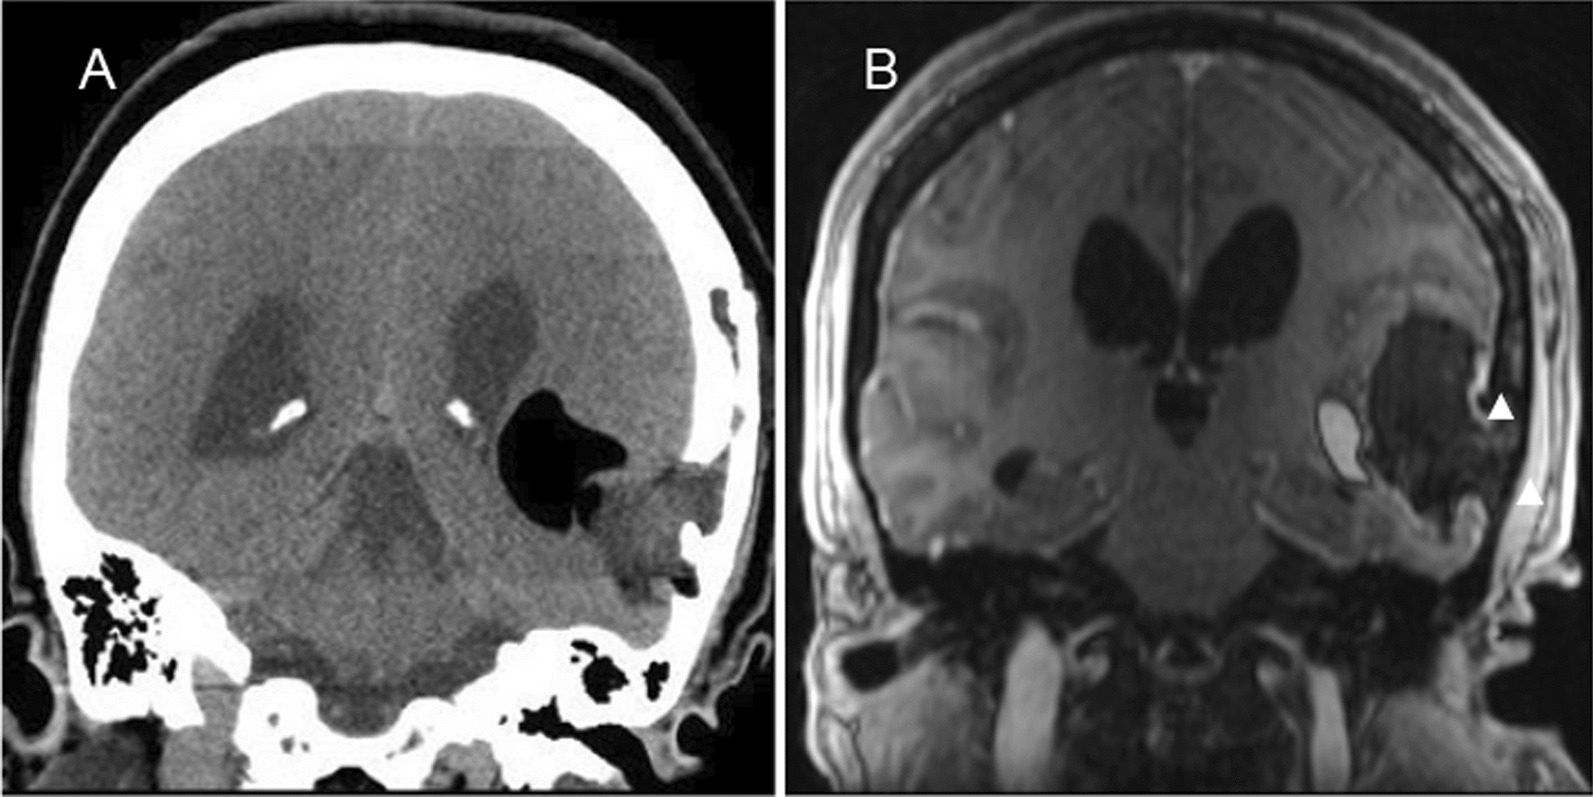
A Computed tomography of head. Left-sided fat-density tumor with erosion into the bone and the presence of a fistulous sinus tract. B Brain magnetic resonance imaging—T1 flair post contrast. Left temporal mass with subcutaneous extension and tiny fat droplets (arrowheads) in the subarachnoid space
